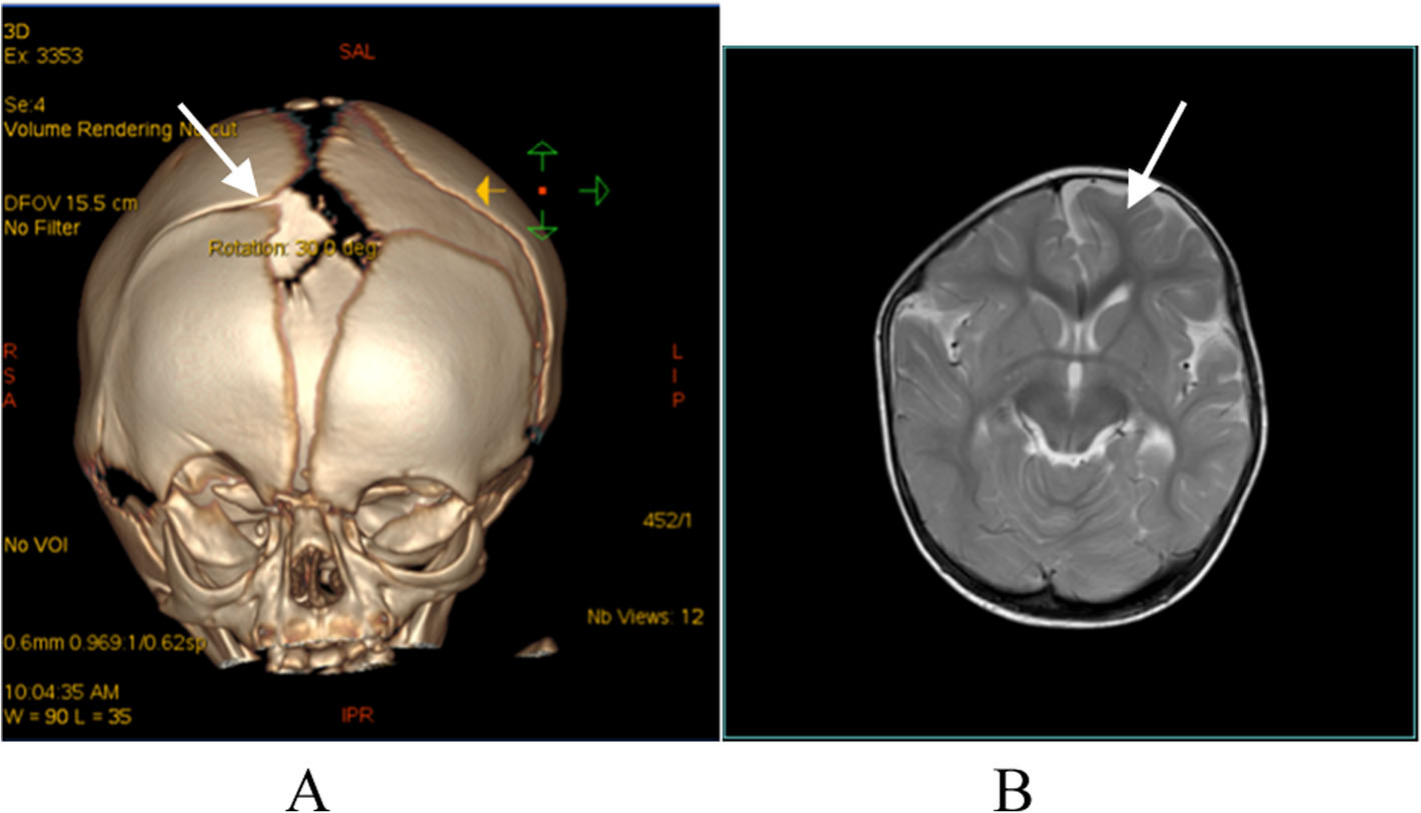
a Three-dimensional reconstruction of a computed tomography (CT) scan of a child’s skull with right UCS after craniofacial surgery. The white arrowhead refers to the right coronal synostosis. 7-b: Cerebral magnetic resonance imaging of another child with right UCS
